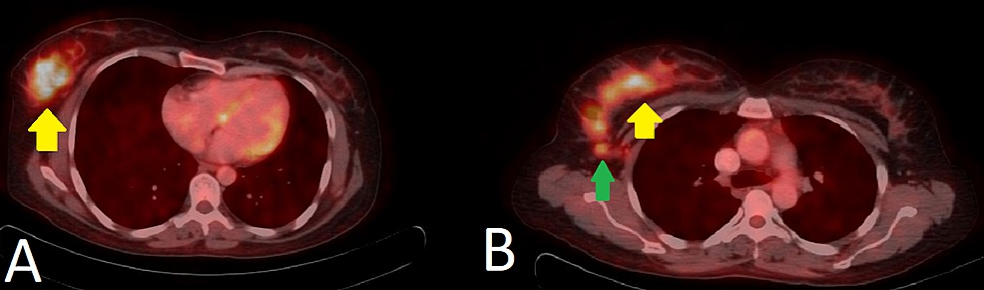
PET-CT scan (axial fused images) Hypermetabolic right breast mass (yellow arrows in A and B) with hypermetabolic axillary lymph nodes (green arrow in B).
